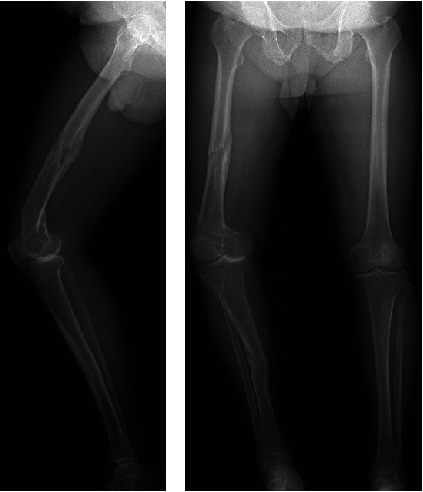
Preoperative radiograph. (a) Lateral view: Femoral deformity is 25° antecurvatum; tibial deformity is 7° recurvatum. (b) Anteroposterior view: Hip–knee–ankle angle is 21.7° varus, femoral deformity is 7° valgus, and tibial deformity is 7° valgus.
